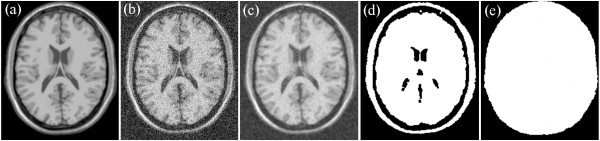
An example of extracting background from T1-weighted MRI. (a) the original T1 weight MRI, (b) the MRI with Rician noise, (c) the result of the median filtering, (d) the result of the morphological close operation, and (e) the result of the brain region.
